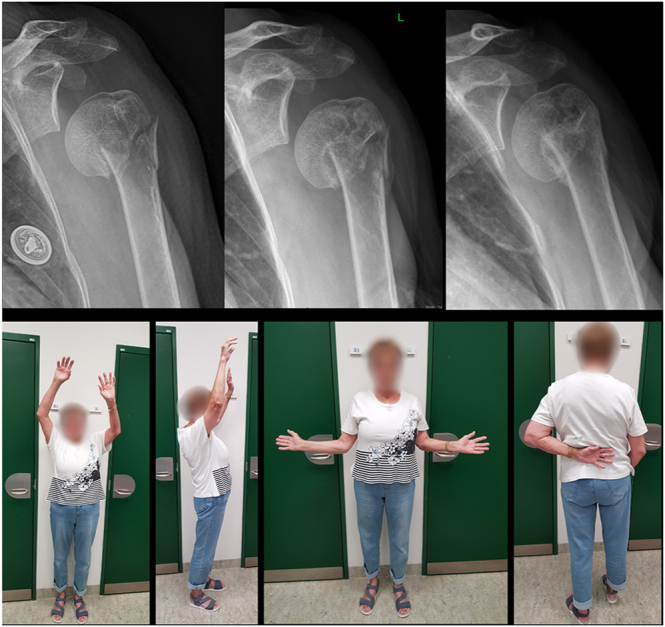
An 80-year-old female presented with a varus-impacted fracture of the surgical neck with a lack of medial support. After 6 weeks, clinical healing was achieved despite incomplete radiographic healing. By 6 months, the humeral head was better centered, and the patient regained pre-injury shoulder function. Anterior–posterior radiographs were taken at 2 weeks, 6 weeks, and 6 months. Photographs were taken at 6 months.
